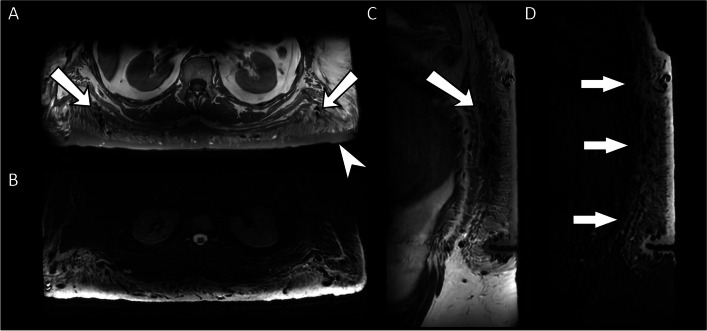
A Coronal T1W MR image in a patient with macrodystrophia lipomatosa demonstrating lipofibromatous hamartoma of the lateral digital nerve of the great toe (arrow). B An axial PD-weighted MR of the ankle in a patient with neurofibromatosis type 1 demonstrates similar thickening of the neural bundles and Schwann cells of the posterior tibial nerve (arrow) as well as multiple extra vessels (arrowheads) in the hypertrophied fat of Kager’s fat pad
